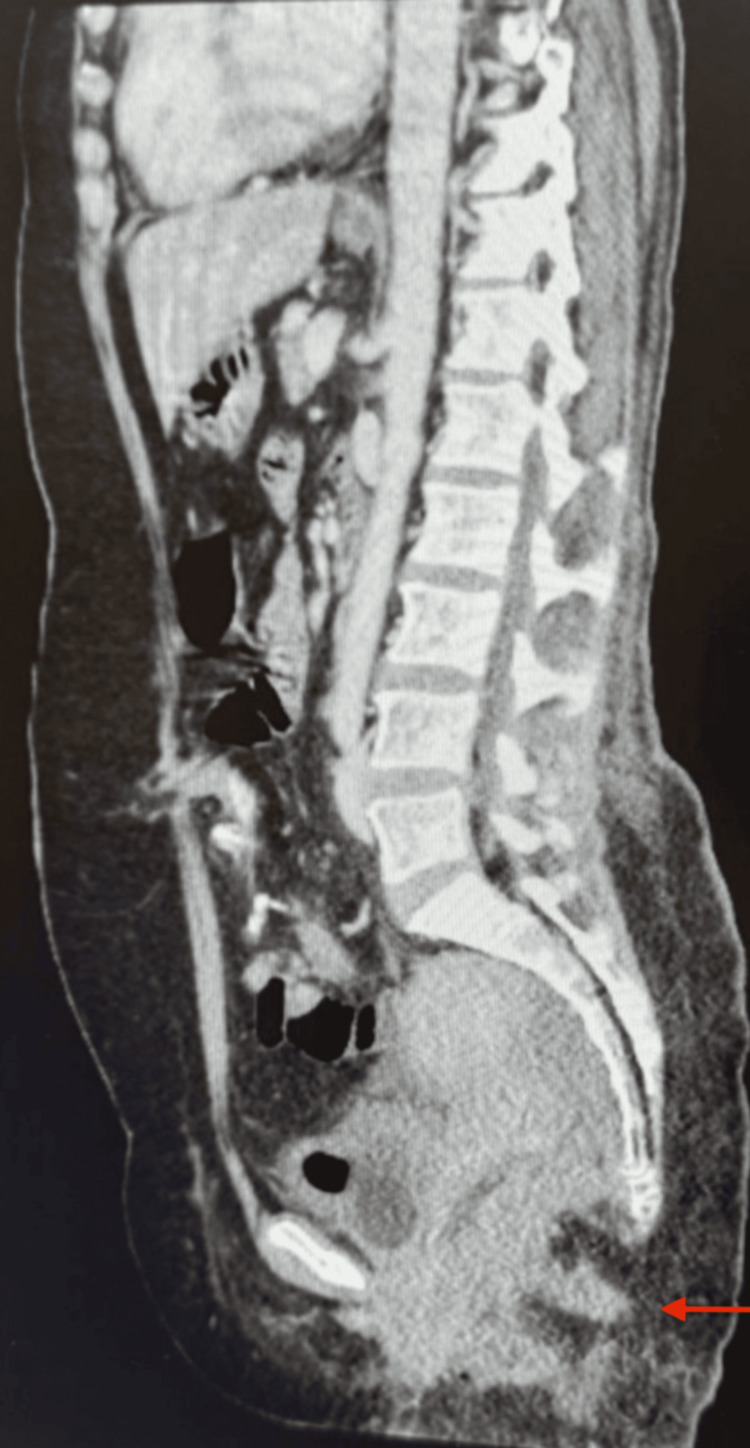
Post-surgery CT of the abdomen and pelvis with IV contrast shows no definitive fluid collection, no bowel obstruction, and unremarkable abdominal organs, with no significant lymph nodes or any vascular concern.
